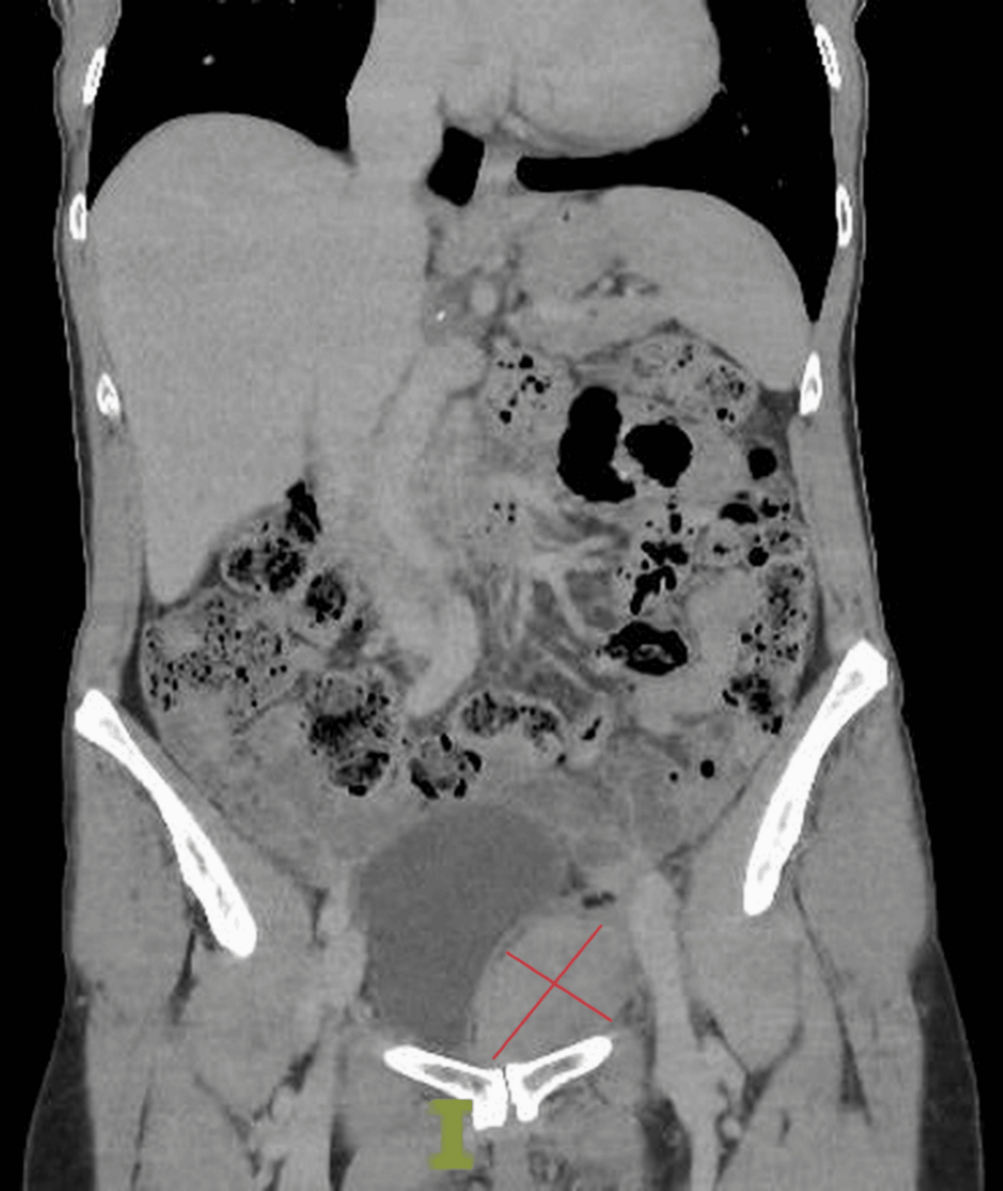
Coronal spiral CT scan with arterial and portovenous contrast phases showing 10 x 4 cm left pelvic haematoma
